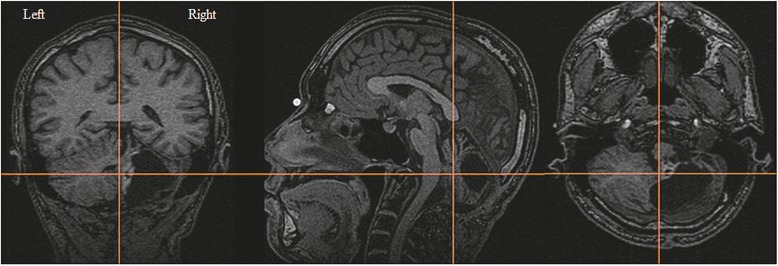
Brain MRI T1-weighted images of the patient. The positions of the coronal, sagittal, and axial images are indicated by the orange lines. A mural nodule of the pilocytic astrocytoma is shown near the crossing point of the orange lines on the coronal and axial images. A cyst associated with the tumor occupies most of right cerebellum
